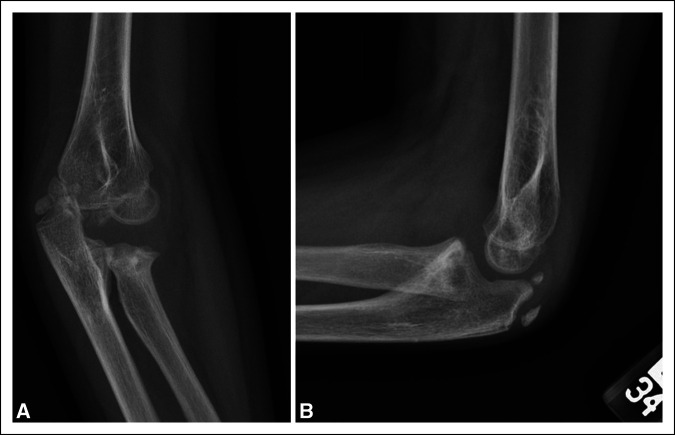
Radiographs of AP and lateral views of the left elbow showing moderate effusion at 1-year mark.
